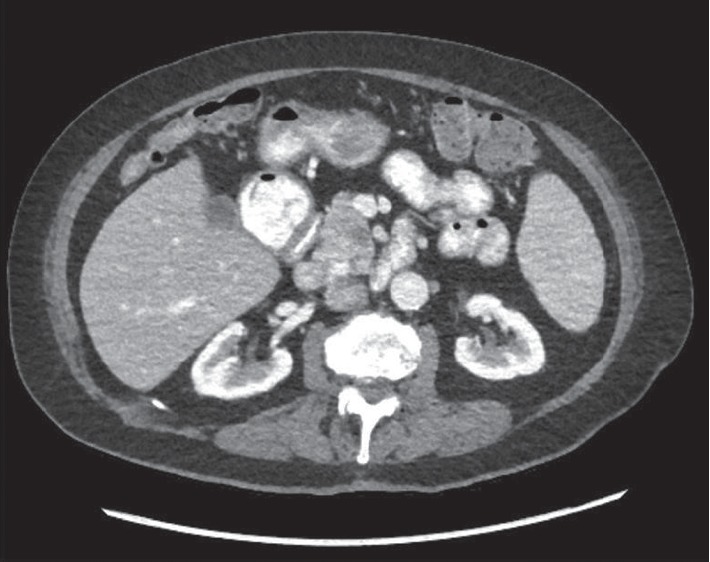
CT abdo/pelvis: normal-appearing pancreas.
